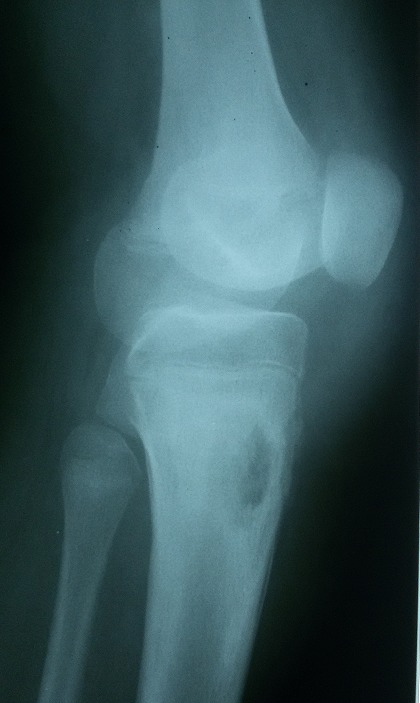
Radiographie du genou. Mise en évidence d'une ostéolyse géographique métaphysaire à limite flou de type Ic de lodwick avec une rupture corticale et une réaction périostée compacte
